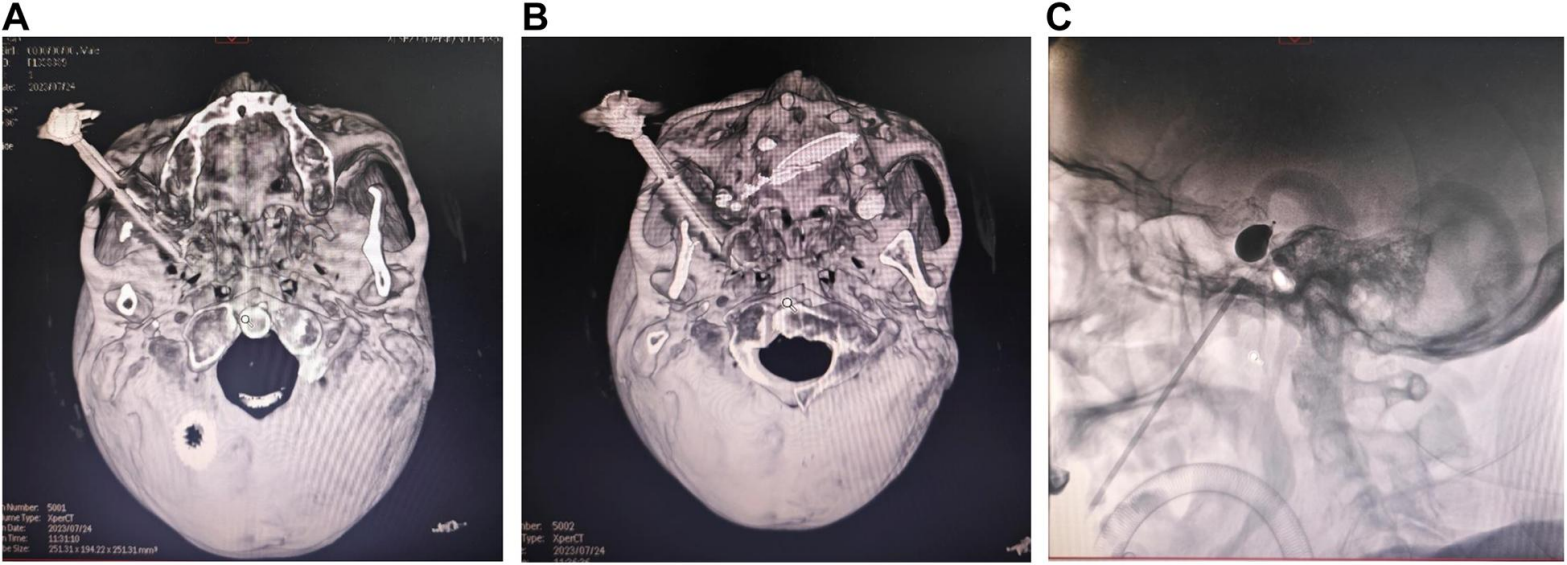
A case involving the adjustment of the puncture needle during surgery. (A) During the operation, the trocar failed to puncture the foramen ovale, and the 3D reconstruction of the acquired CT images of the skull base indicated that the trocar was displaced. (B) The trocar's position was readjusted, and a subsequent 3D reconstruction of the acquired CT images of the skull base revealed that the trocar was precisely punctured into the foramen ovale. (C) Following balloon inflation, the lateral cephalometric image displayed a typical pear shape.
